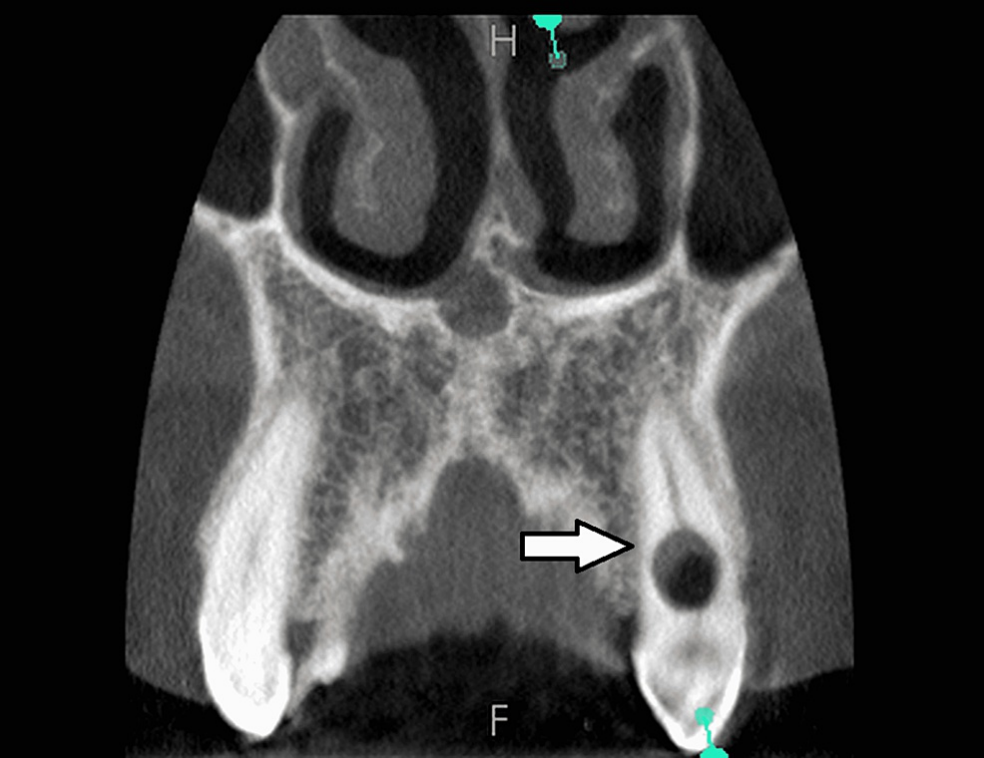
Cone-beam computed tomographic scan of the resorption site
